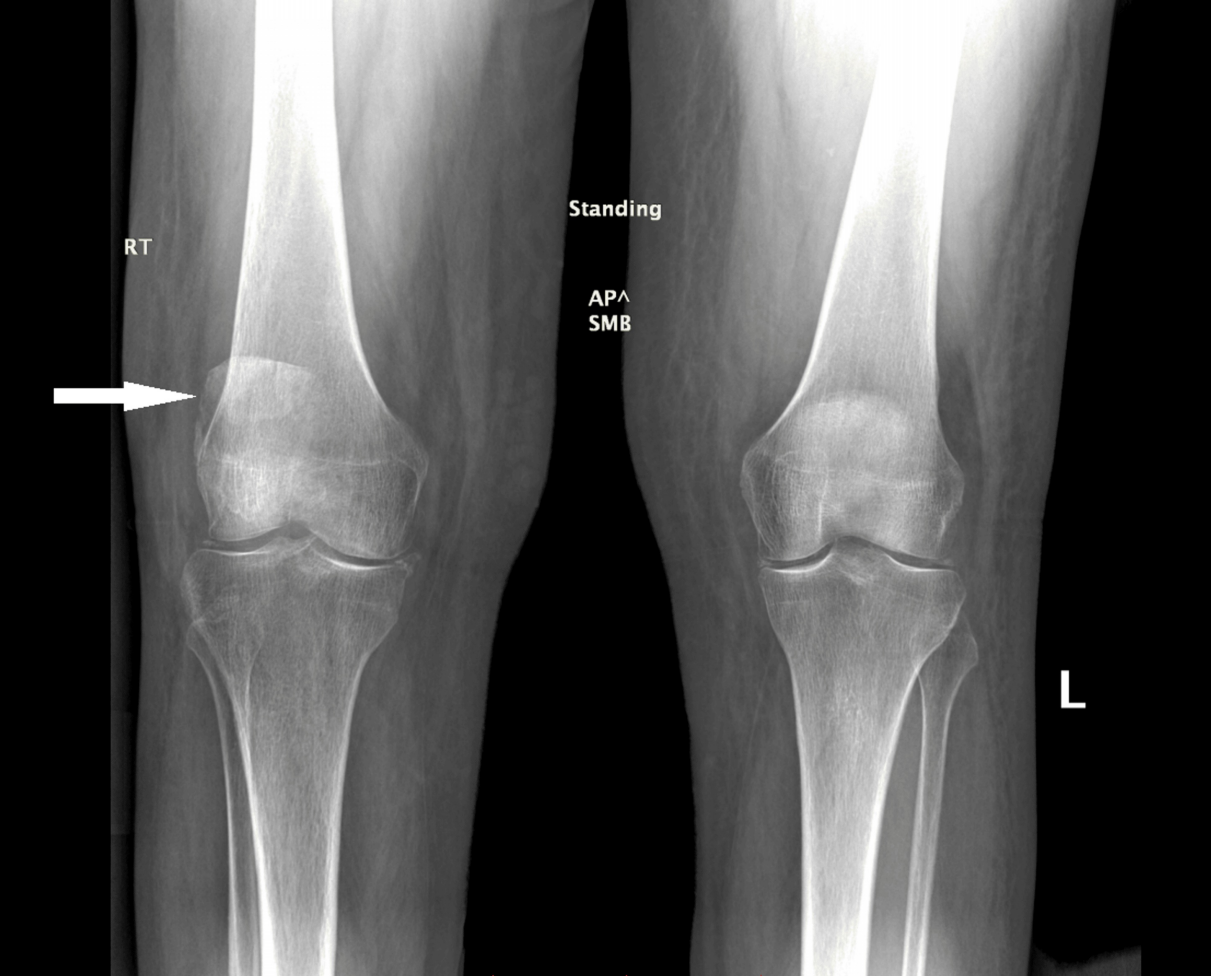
Coronal view X-ray of both of the patient's knees showing the superior displacement of patellar fragments of the right knee compared to the intact left knee.
